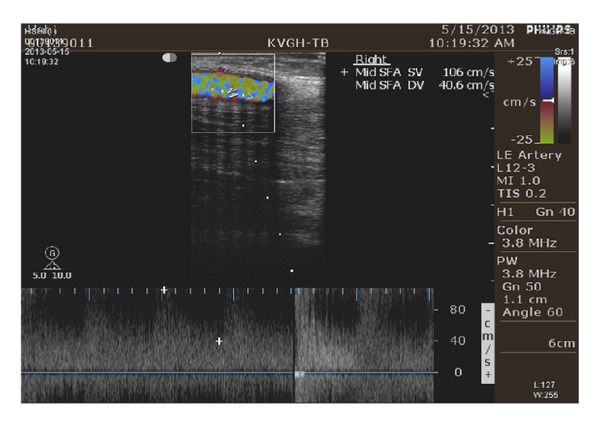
Ultrasonic image examination date: May 15, 2013, Kaohsiung Veterans General Hospital, Tainan Branch.
